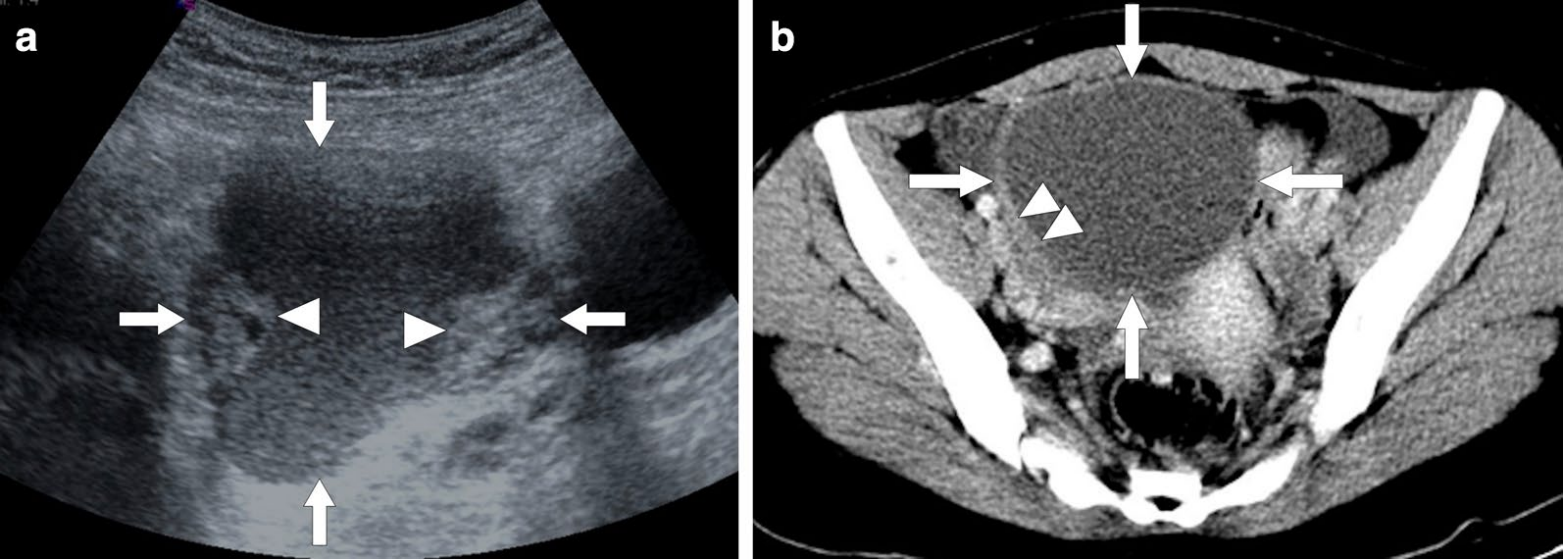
Pelvic endometriosis. A 47-year-old woman with recent onset of pelvic pain and fullness. Gray scale US image a showed a large cystic mass (arrows) with solid-appearing mural nodules (arrowheads). There was no apparent blood flow on color Doppler imaging. Axial plane postcontrast CT image b shows the same cystic lesion (arrows) with asymmetrically thickened enhancing wall (approximately 45 HU) (arrowheads). Surgical resection confirmed endometrioma with no evidence of malignant component
